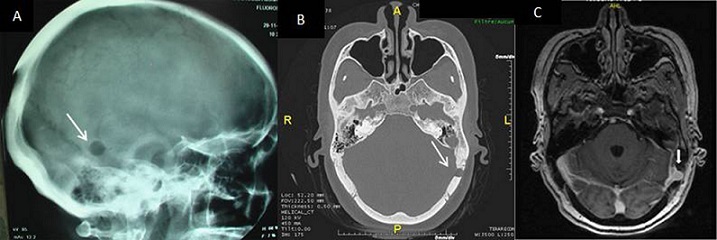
(A) radiographie du crane: lacune en regard de l'os temporal gauche; (B) TDM du massif facial: image lacunaire de 9 mm à l'emporte pièce de l'os temporal gauche; (C) IRM en coupe axiale T1 Gado: lacune en regard du sinus transverse gauche avec lequel elle se continue et se rehausse de la même façon que le sinus après injection de gadolinium
